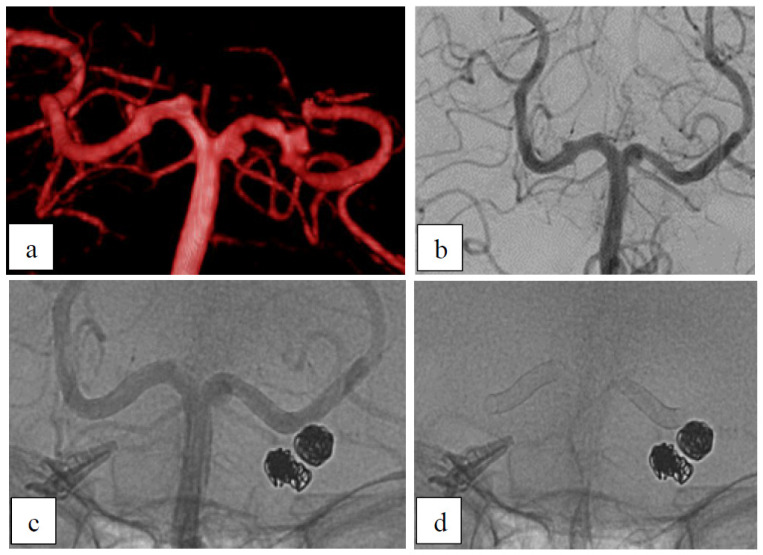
Dysplastic aneurysms. (a) Pre-interventional 3D angiogram showing two dysplastic aneurysms of both P1/P2 segments. (b) After 3 months, there is no residual inflow into the aneurysm of the left P1/P2 segment. The dysplastic aneurysm of the right P1/P2 segment remained. (c) Postinterventional non-subtracted angiogram. (d) X-ray showing the implanted p48 HPC device in the P1/P2-segments. There is also a clip and two aneurysms of the anterior circulation packed with coils.
